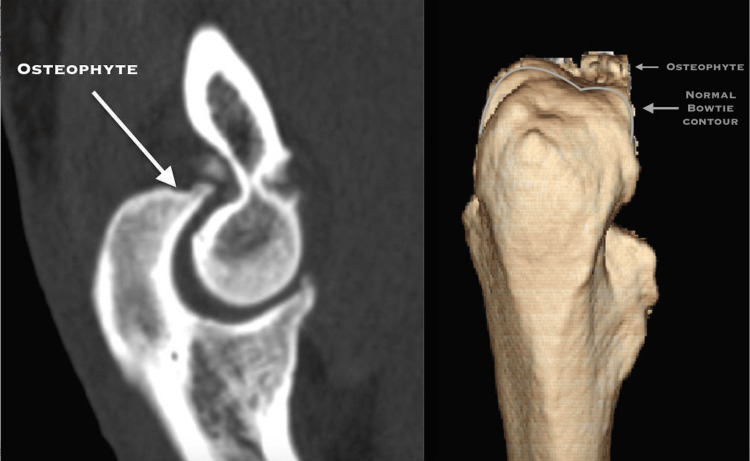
A preoperative CT scan of the elbow locating the impinging osteophytes Original work of authors
